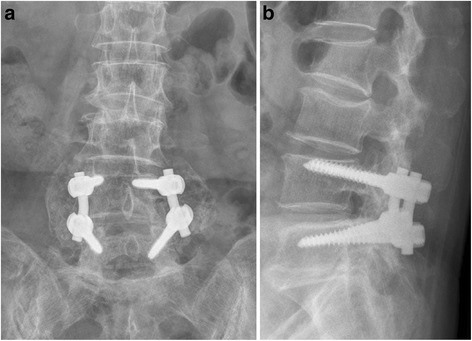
Post-fusion radiographs of a 64-year-old woman showing a good fusion mass at 14 years and 7 months of follow up. a anteroposterior view. b lateral view
